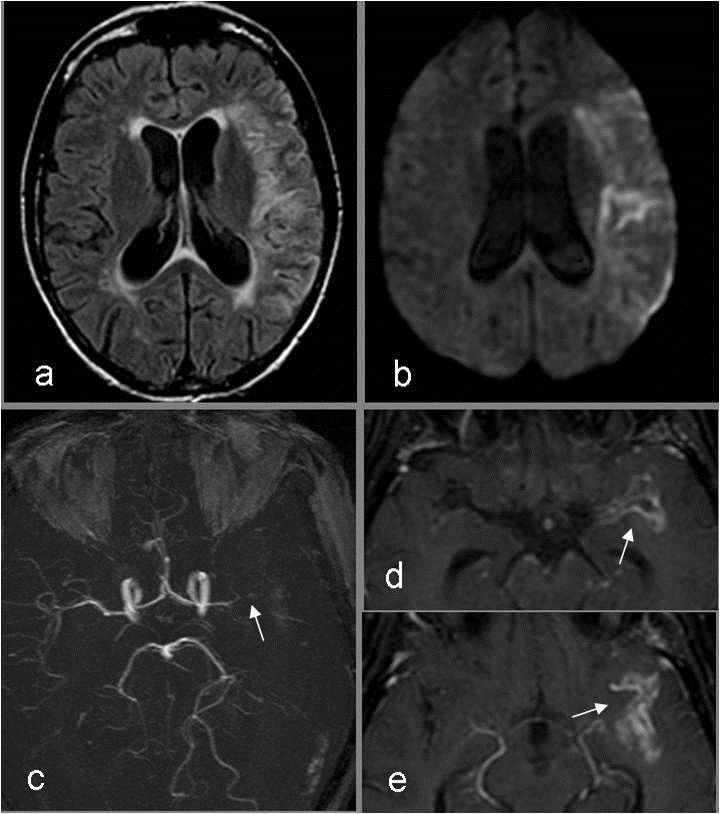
a) MRI fluid attenuated inversion recovery (FLAIR): temporal-parietal left side cortical sub-cortical hyper intense lesion; b) MRI diffusion weighted images (DWI): reduced diffusion consistent with recent ischemia; c) angio MRI: absence of flow through the left middle cerebral artery (arrow), d and e) thickening and contrast enhancement on the left Sylvian fissure surrounding the cerebral artery.
